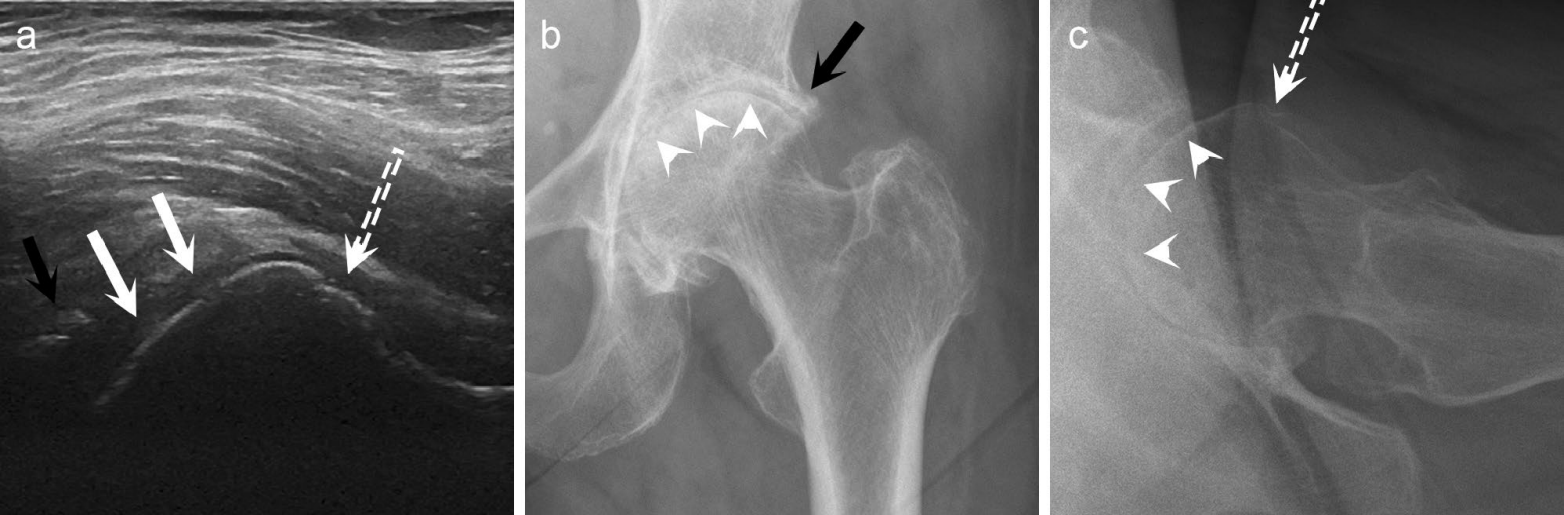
Ultrasonography (US) detects deformity of the femoral head better than conventional radiography (CR). In longitudinal US view of the hip joint (a), marked deformity of the femoral head is seen (white arrows), whereas on anteroposterior (b) and lateral CR views (c) the femoral head has preserved its normal ball-like appearance (white arrowheads). Additionally, a large femoral acetabular osteophyte (black arrow) is observed on US (a) and anteroposterior CR (b). Furthermore, femoral collum osteophyte (dashed white arrow) is seen on US (a) and on lateral CR (c).
